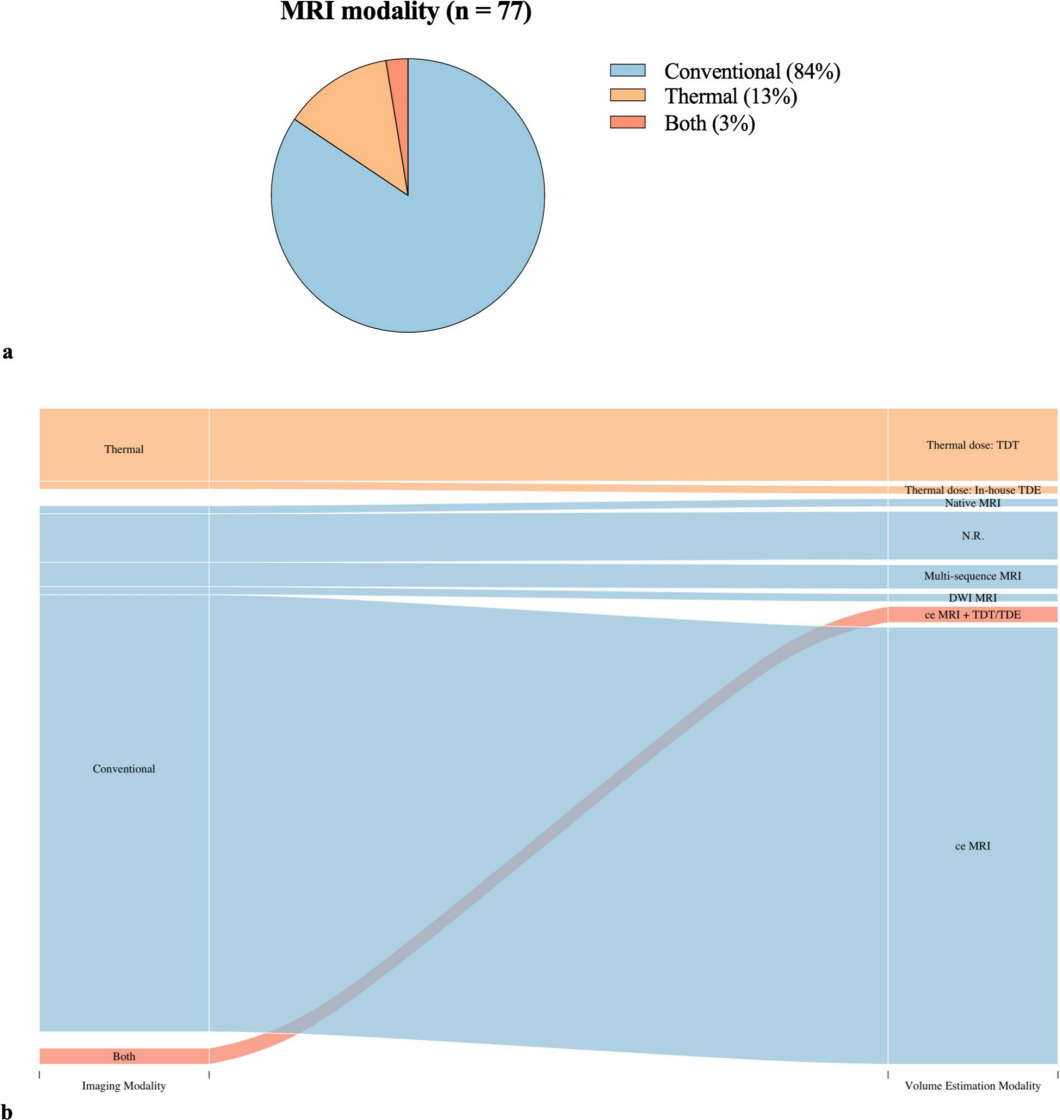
Imaging modalities (2a) and volume estimation modalities applied for first volume assessment (2b) after LITT. Abbreviations: N.R. = not reported, TDT = Thermal Dose Threshold, TDE = Thermal Damage Estimate, DWI = diffusion-weighted imaging, ce = contrast-enhanced
